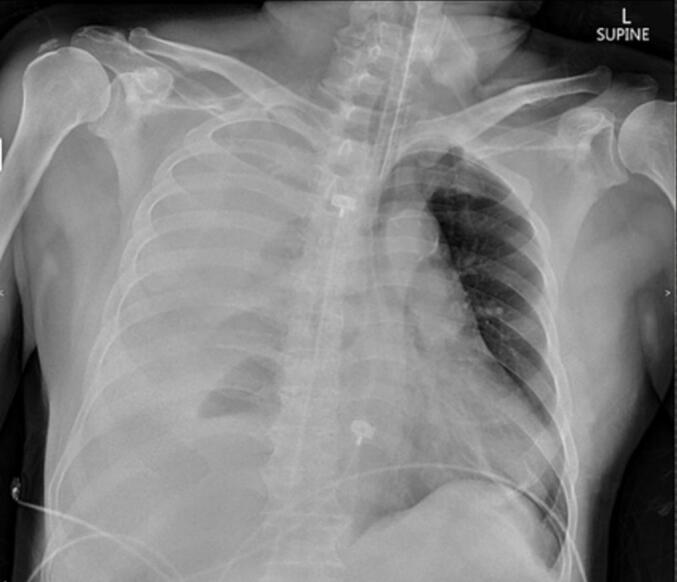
Chest X-ray showing a large amount of right haemothorax.
